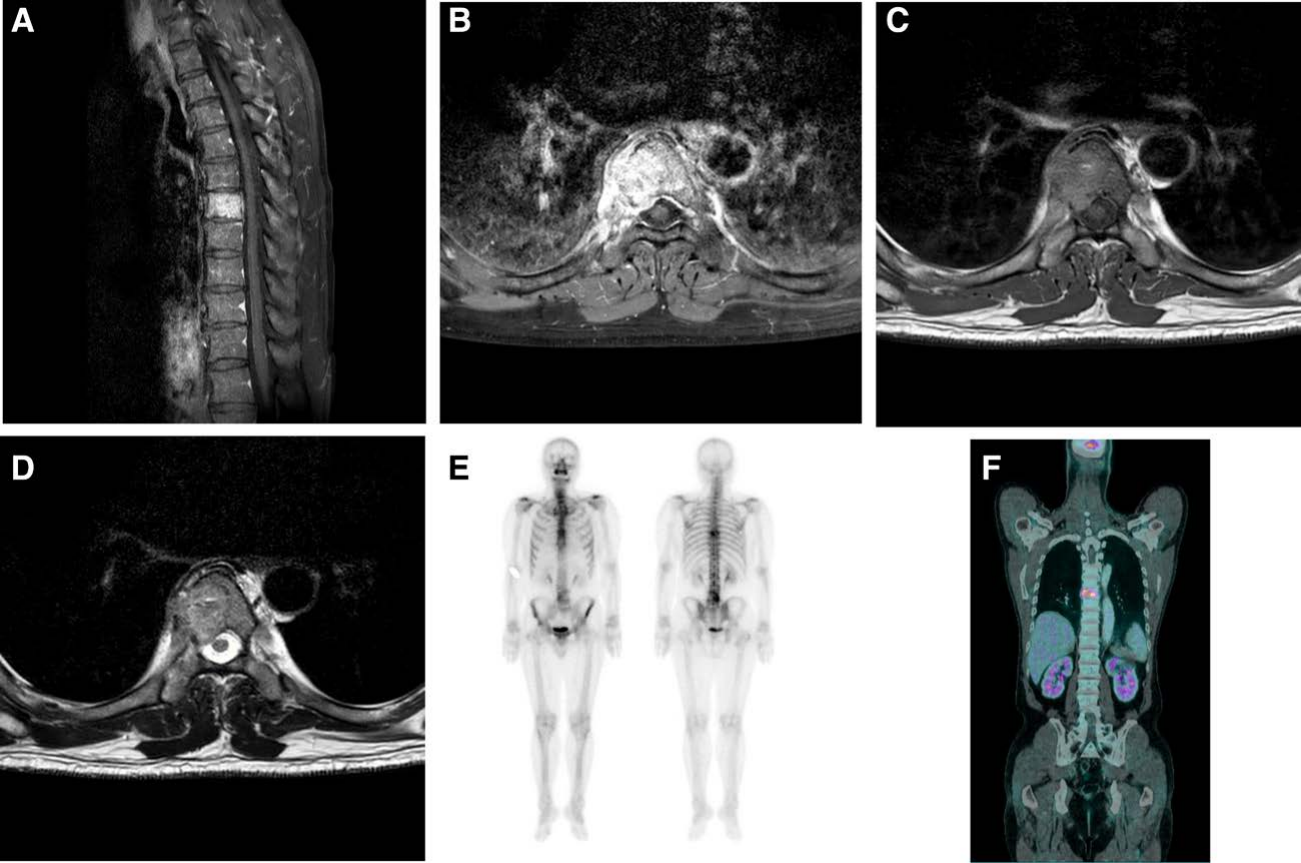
Magnetic resonance imaging (MRI): A and B are coronal and axial T1-weighted images after gadolinium administration, showing neoplastic involvement of the T7 vertebral body. C and D show grade II epidural spinal cord compression. Bone scan and PET CT: E and F show T7 involvement. PET CT = positron emission tomography/computed tomography.
